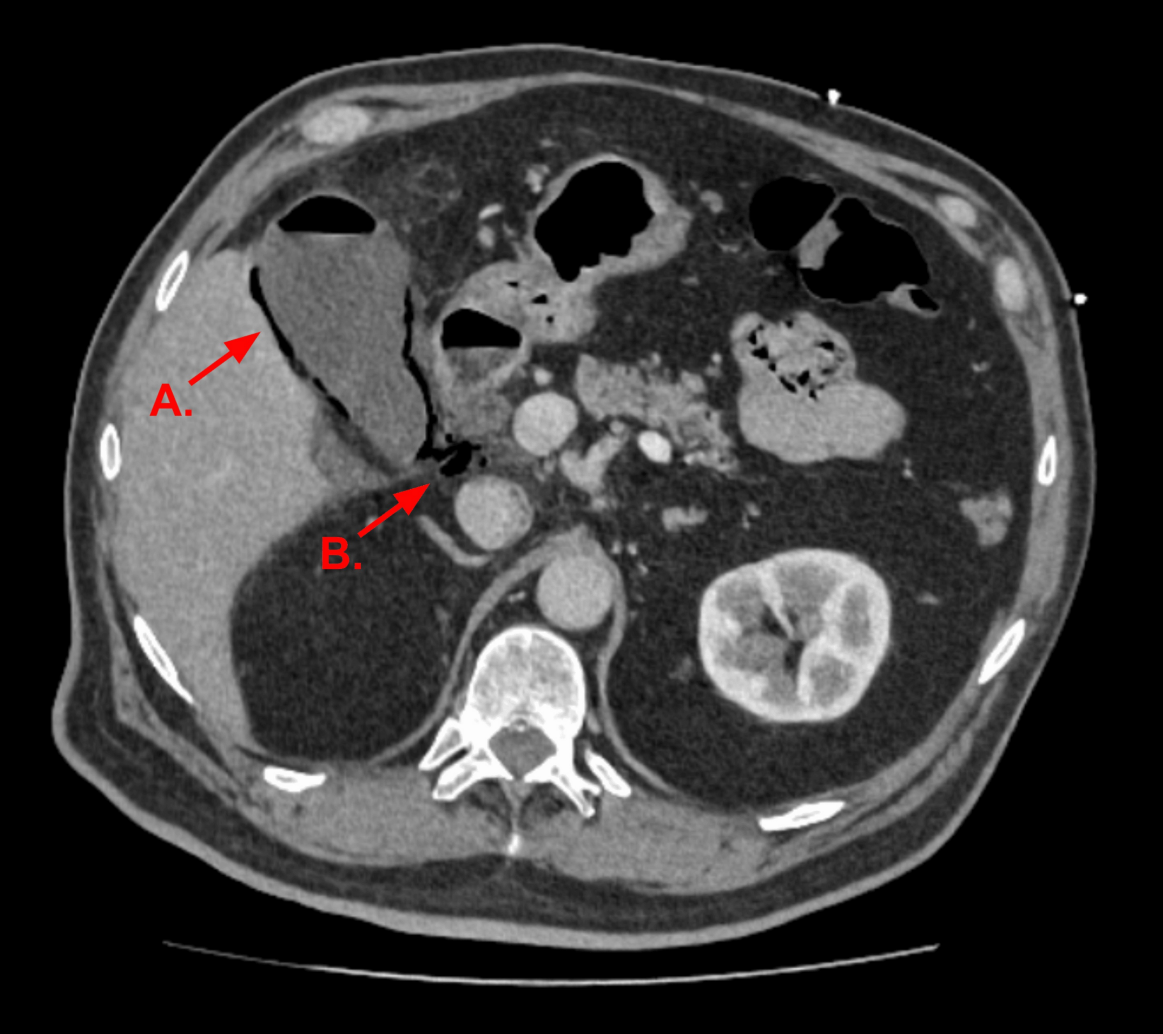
CT Abdomen and Pelvis With Contrast. The gallbladder wall demonstrated extensive gas content (A) associated with small pneumobilia. A few locules of pneumoperitoneum were also visible in the upper abdomen (B).
